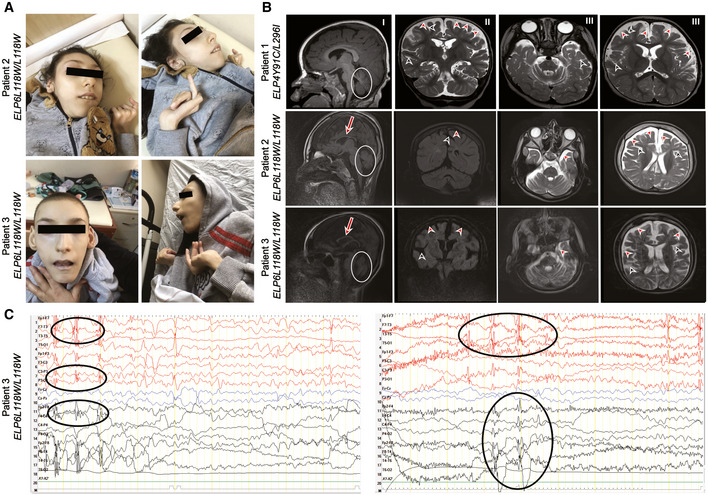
Clinical information of the patients with neurodevelopmental disorders and identified *ELP4*/*6* variants Anterior and lateral photographs of two patients with *ELP6* variants showing dysmorphic features at 20 (Patient 2) and 19 (Patient 3) years of age.Mid‐sagittal (I), coronal (II) and transversal (III) MRI scans of Patient 1 with newly identified *ELP4* variants and Patient 2 and Patient 3 with *ELP6* variants. Atrophy of the cerebellum with prominence of the cerebellar folia is marked by circles. Arrows indicate thin corpus callosum, red arrowheads point to enlarged extra‐axial CSF spaces, and black arrowheads to cerebral sulci in keeping with cerebral and cerebellar volume loss.EEG of Patient 3. Left panel: 1–2 Hz left fronto‐temporal spike and multi‐spike slow wave discharges (Fp1‐F7 and T3‐T5) spread to the homologous same hemisphere and opposite hemisphere, predominantly seen on bipolar montage EEG. Right panel: Spike‐ and multi‐spike slow wave activities together with fast rhythms originating from bilateral fronto‐temporal‐frontocentral regions indicate electrographic seizure activity. Described changes are marked by circles.
